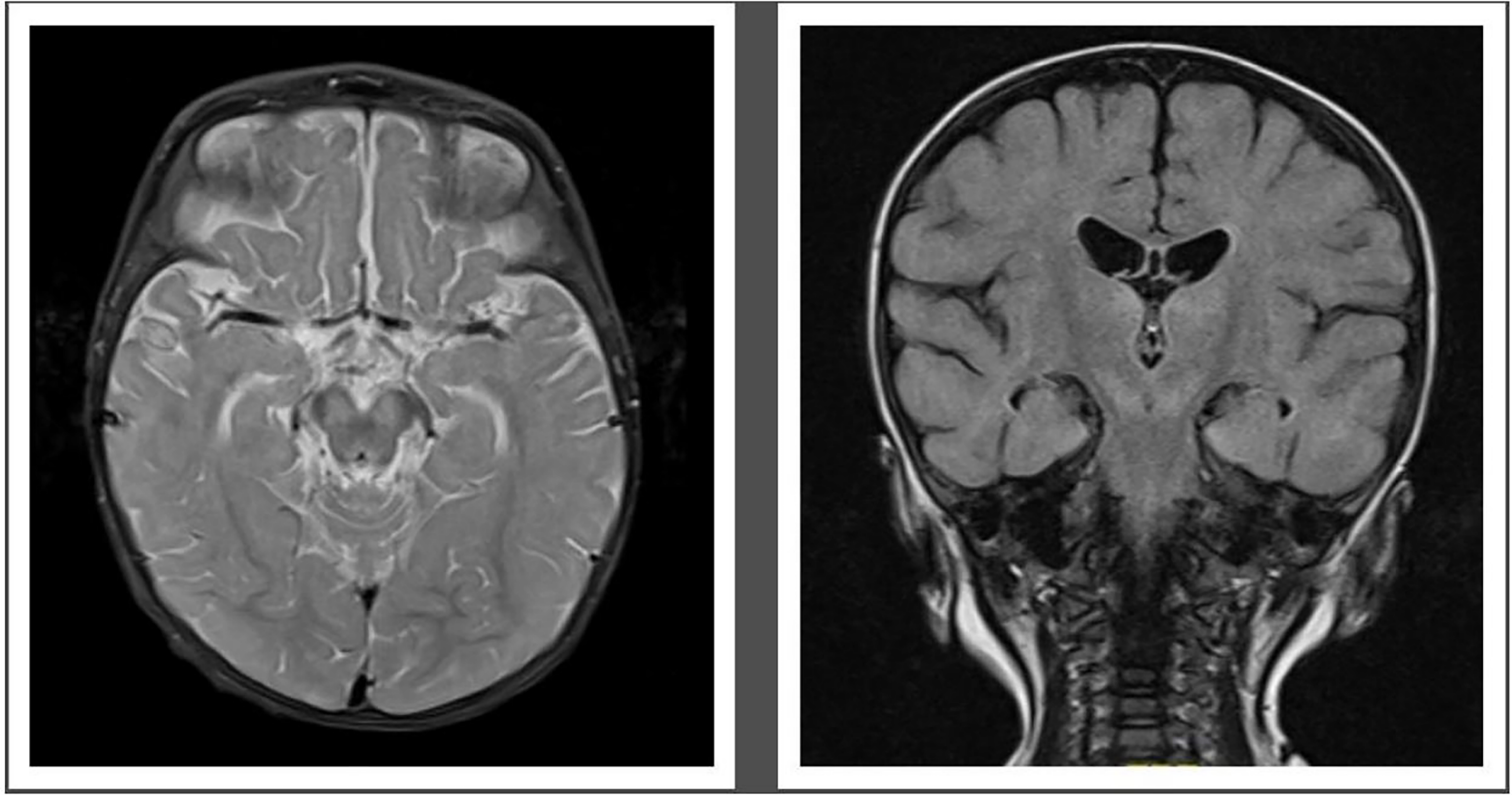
Pretreatment MRI-Brain demonstrating T2 hyperintense signal in midbrain (left) and hyperintense Fluid attenuated inversion recovery (FLAIR) signal in both thalami and midbrain (right).
